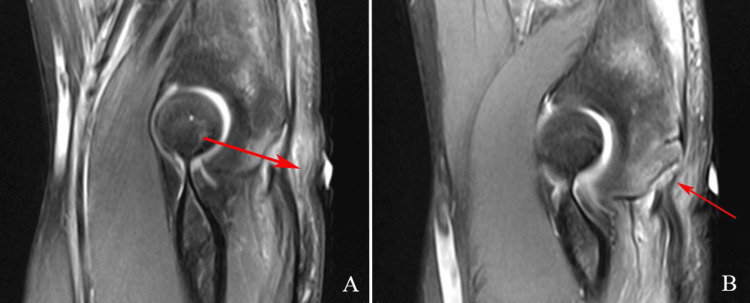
Magnetic resonance images (A) and (B) demonstrating acute full-thickness avulsion of the distal triceps tendon occurring at the olecranon enthesis.
